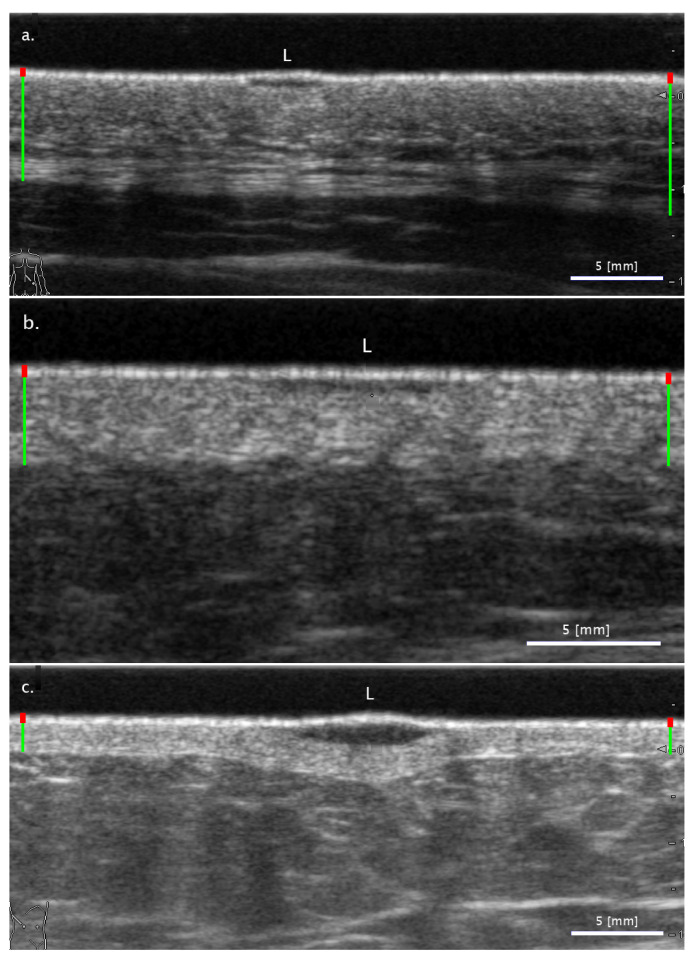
Representative ultrasound images of the three examined lesion types. (a) Nevus; (b) BCC; (c) MM. The primary layers of the skin are marked by the red (epidermis) and green (dermis) marks on the sides of the images. The lesions are marked by a white letter ‘L’ placed above them on the images.
